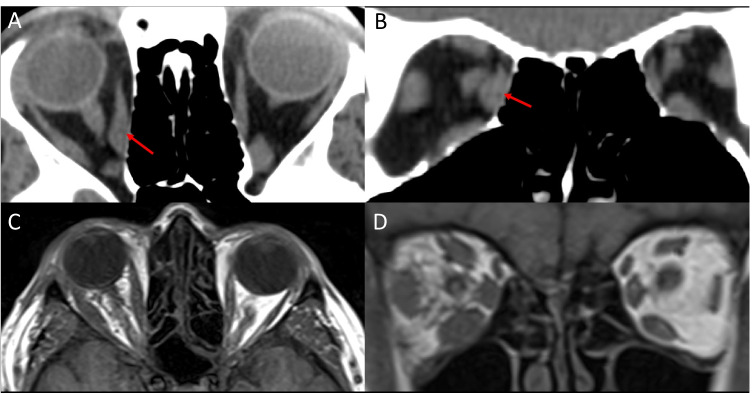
Breast cancer metastasis. Case 1—axial CT image of the orbits (A) demonstrating focal enlargement of the right medial medial rectus (arrow). Coronal CT image (B) demonstrates enlargement of the right medial rectus which is approaching the optic nerve. Case 2—axial T1-weighted MRI (C) demonstrating isointense enlargement of the medial and lateral recti muscles and intraconal fat infiltration. Coronal T1-weighted MRI (D) shows diffuse enlargement of the right extraocular muscles with intraconal fat infiltration
